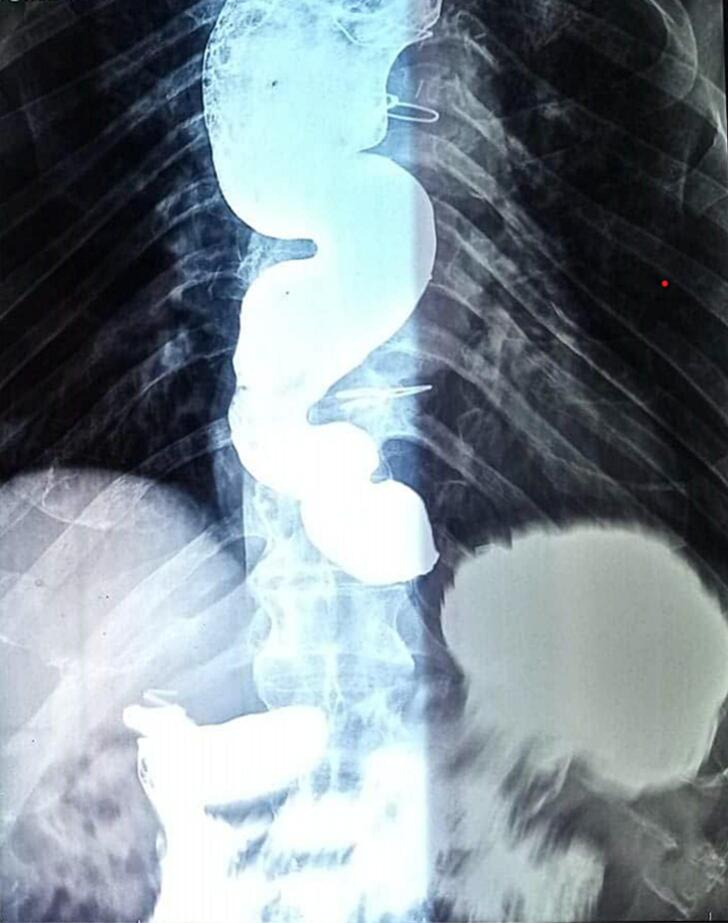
Barium X-ray with a sigmoid shape esophagus.
